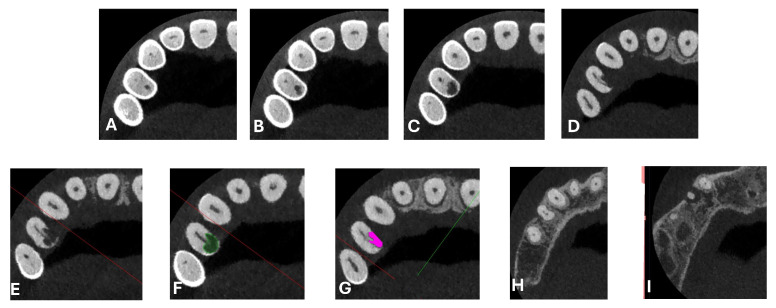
Axial CBCT images of tooth 1.4, showing its ECR and PL. (A–E) Sequential scrolling on the axial plane from the cervical area to the middle third of the root; (F,G) Segmentation of Figure 2E using the advanced software functions iRYS^®^ (MyRay, Imola, Italy); (H,I) Sequential scrolling on the axial plane of the apical third of the root, including the PL.
